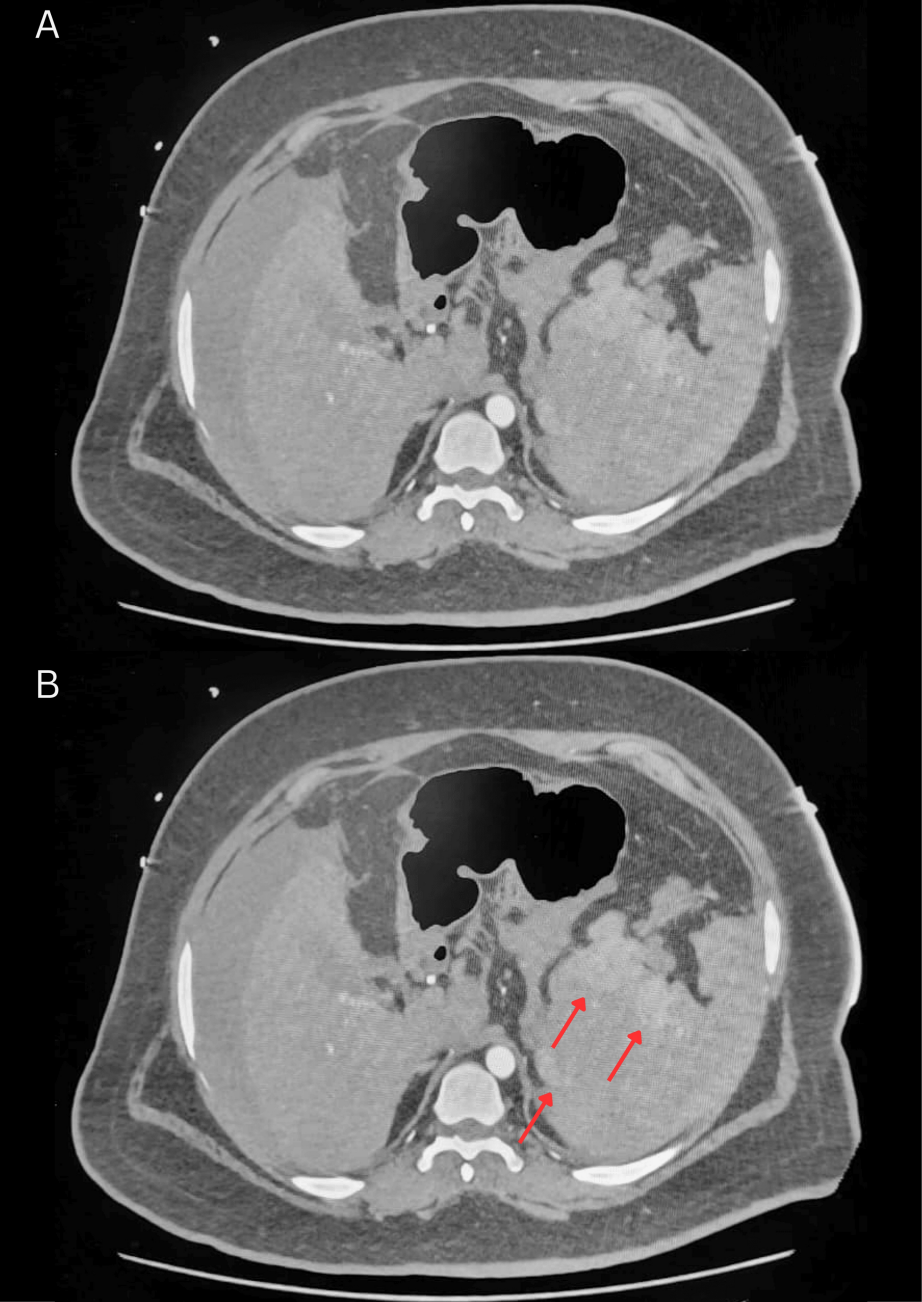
Contrast-enhanced axial arterial-phase CT of abdomen demonstrating splenic rupture Pre-operative axial arterial-phase contrast-enhanced CT suggestive of splenic parenchyma with focal areas of contrast extravasation (red arrows). (A) shows the unannotated scan and (B) shows the annotated scan.
